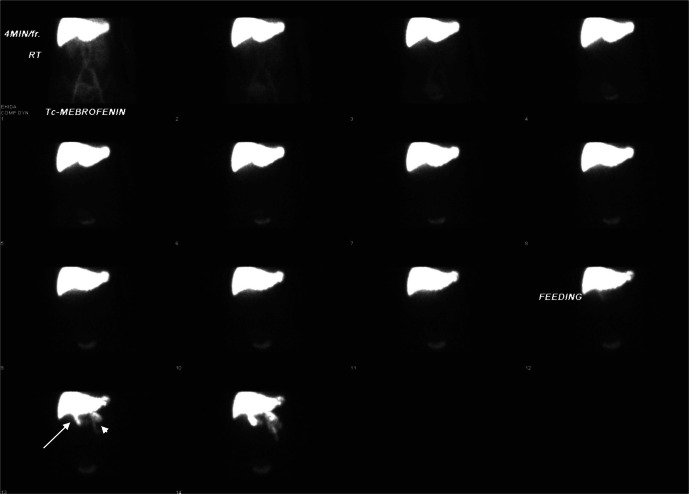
Hepatobiliary scintigraphy showed good hepatic tracer extraction with transit
